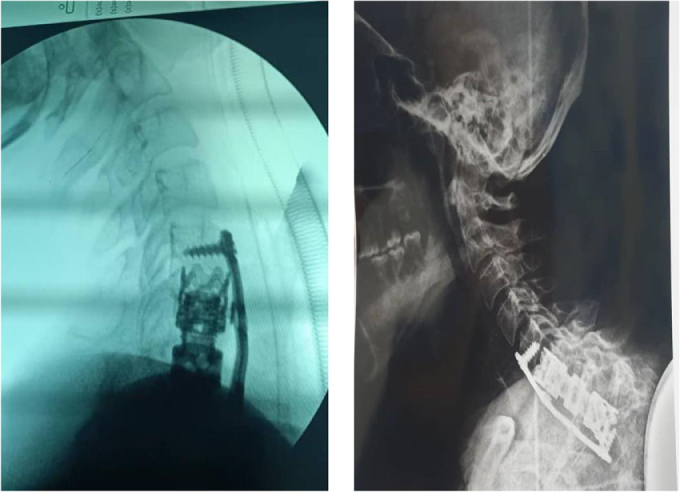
Intraoperative fluoroscopy shows the good alignment of the fixation device (left). Postoperative X-ray (right).
